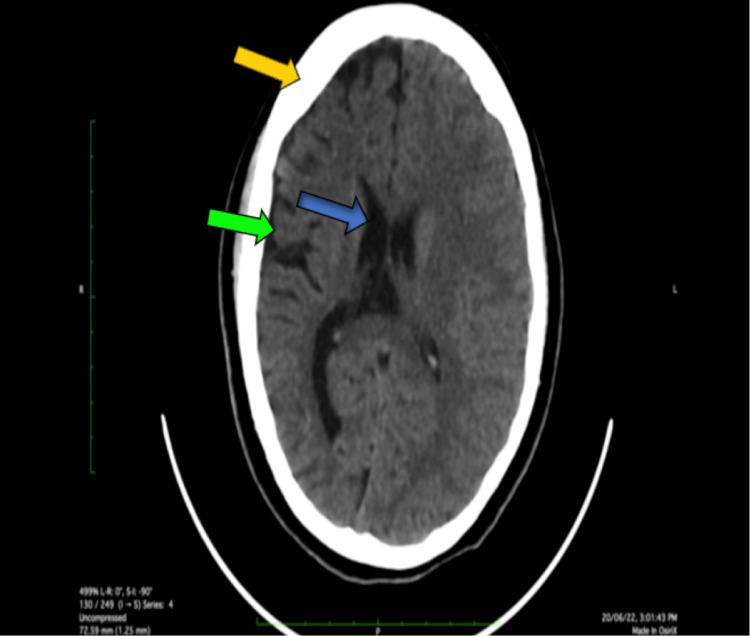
Plain CT images of the brain show atrophy of the right cerebral hemisphere (green arrow) with ipsilateral ventriculomegaly (blue arrow) and ipsilateral calvarial thickening (yellow arrow). CT: computed tomography
